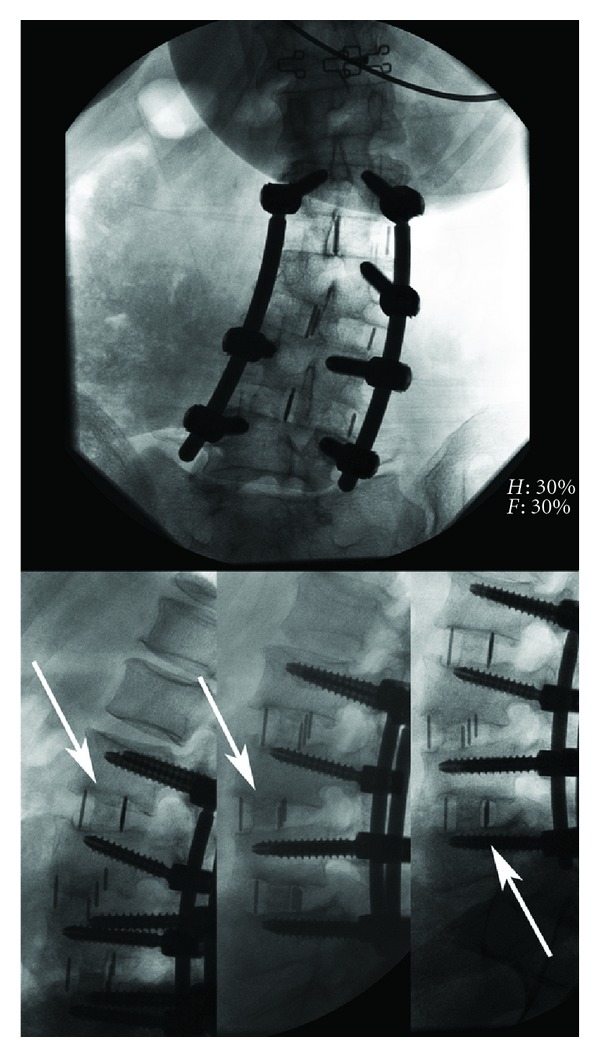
Anterior and lateral fluoroscopy-guided, level-by-level radiography. Note that parallel endplates were obtained on lateral radiography using this technique (white arrows), avoiding X-ray parallax.
